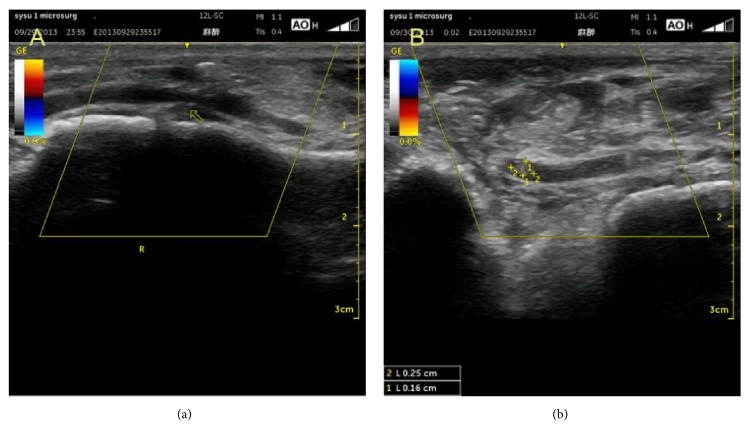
Vascular abnormalities on ultrasonography: decreased arterial diameter and blood flow (a); partial arterial or venous disruption, partial arterial thrombosis (b).
